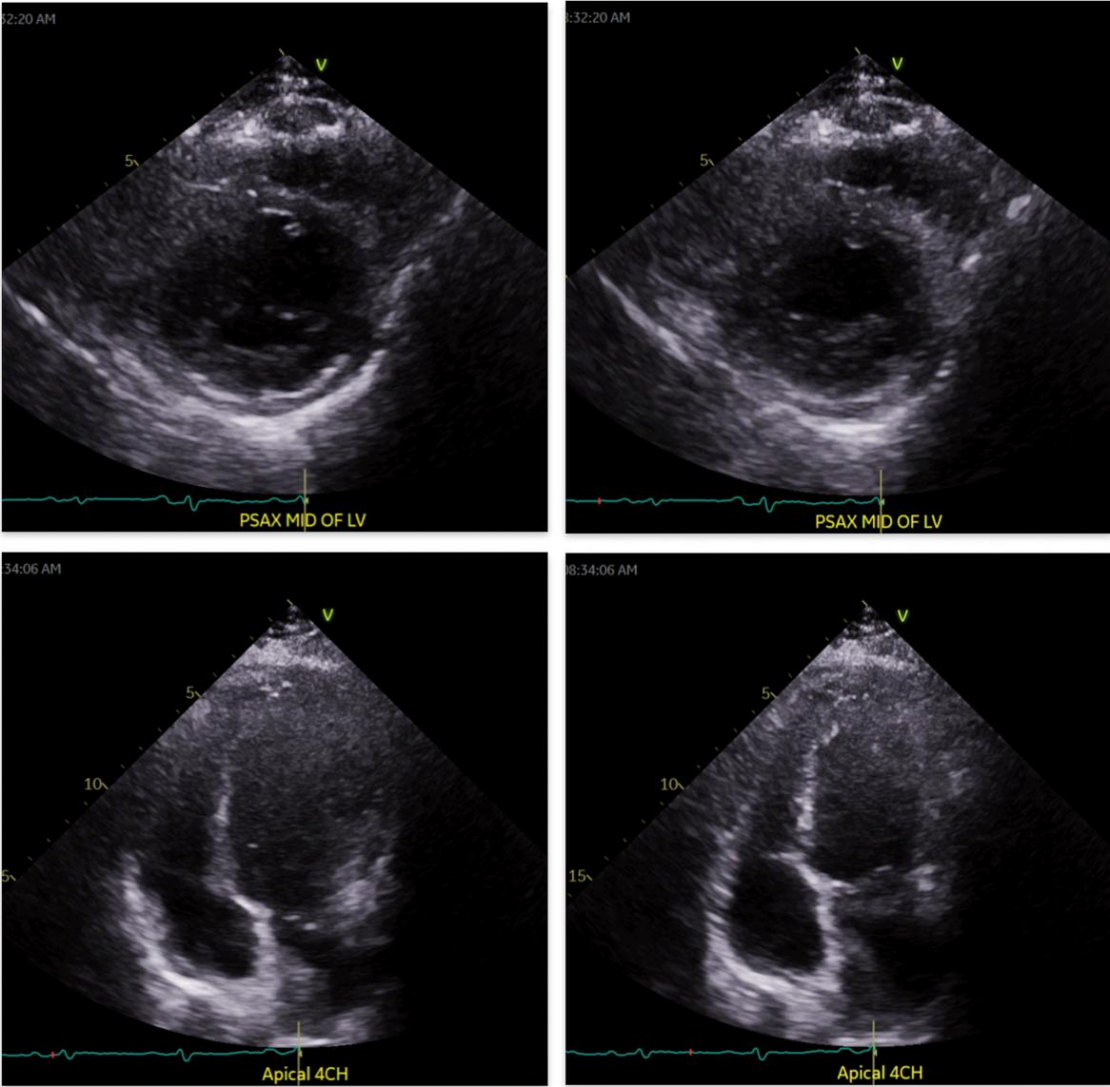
Post-operative echocardiogram showing diastolic (left) and systolic (right) phases with resolution of septal bounce.
